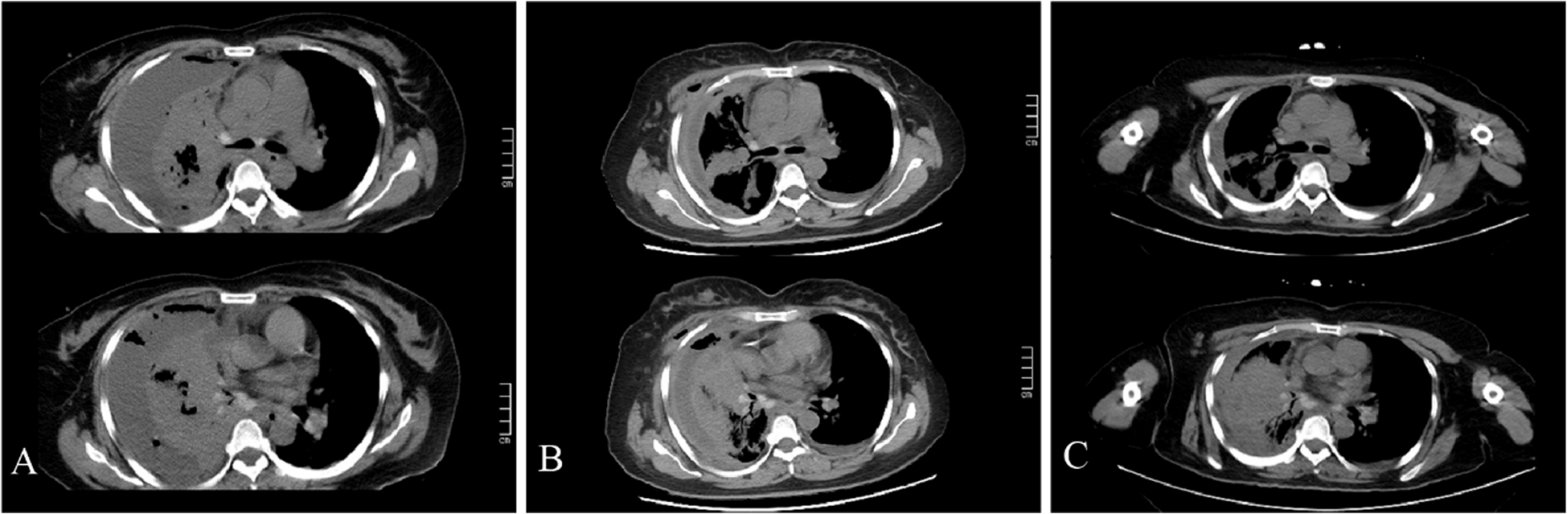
CT showed hydropneumothorax and extensive compression atelectasis on the right side of the chest on November 1st (a). After thorough pleural drainage and anti-infective treatment, CT showed right pleural thickening, pleural effusion, multiple cord shadows in the middle and lower lobe, and consolidation of the lower lobe on November 18 (b). On December 7, CT re-examination showed that the multiple cords in the middle and lower lobe of the right lung were obviously absorbed and the consolidation range of the lower lobe was slightly reduced
